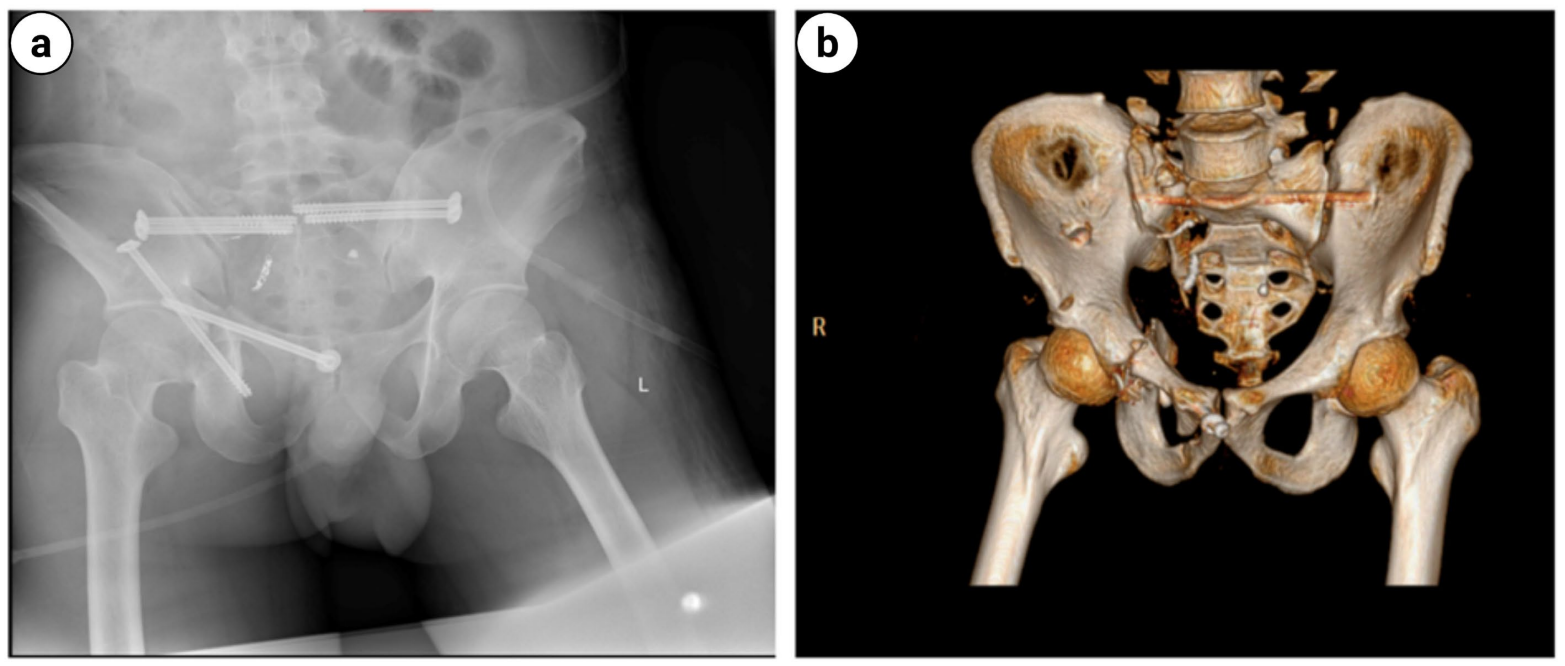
Postoperative day-2 follow-up pelvic radiograph and three-dimensional computed tomography (3D CT) revealed excellent quality of fracture reduction with appropriately positioned screws: (a) Pelvic radiograph; (b) Three-dimensional pelvic computed tomography.
